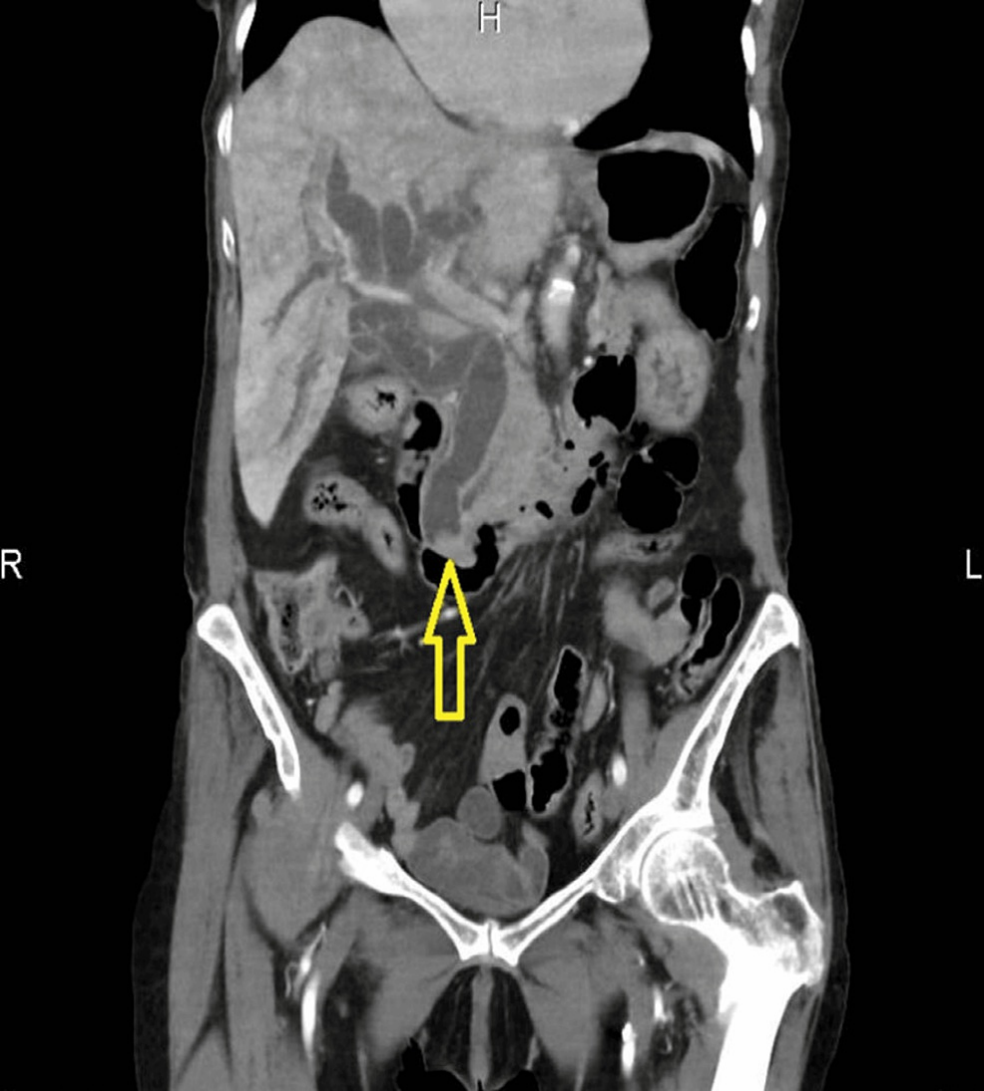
Coronal section of pre-ERCP CT showing dilated CBD and stone at the distal CBD (yellow open arrow). ERCP: endoscopic retrograde cholangiopancreatography; CT: computed tomography; CBD: common bile duct
